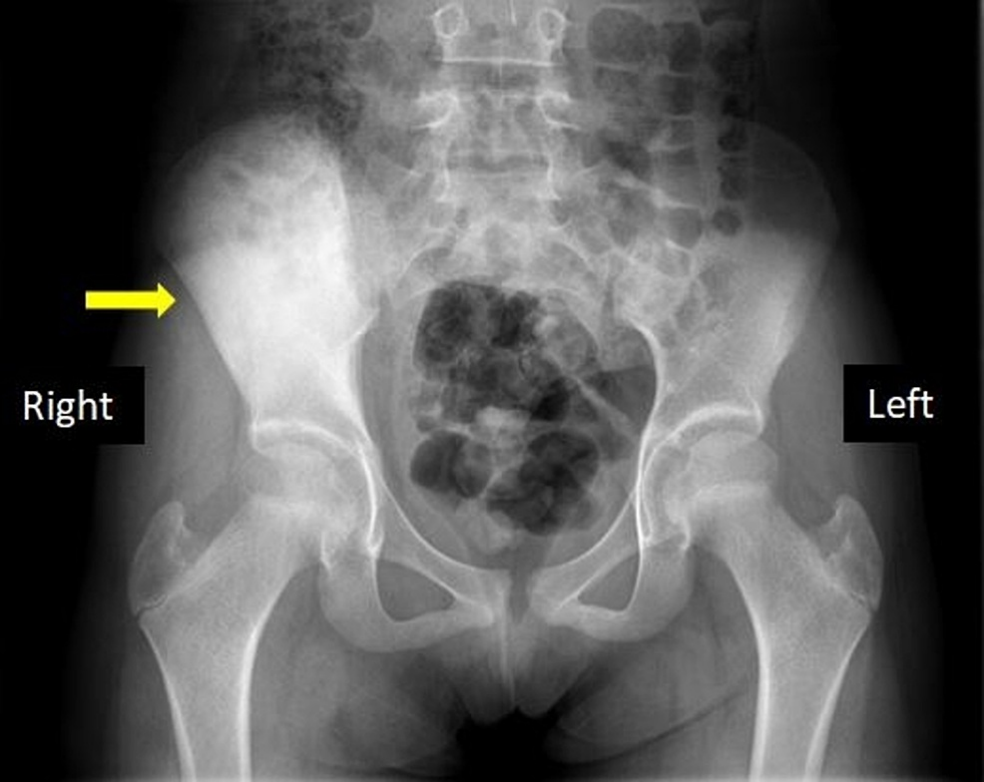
X-ray pelvis. It shows diffusely sclerotic right hip bone (yellow arrow).
